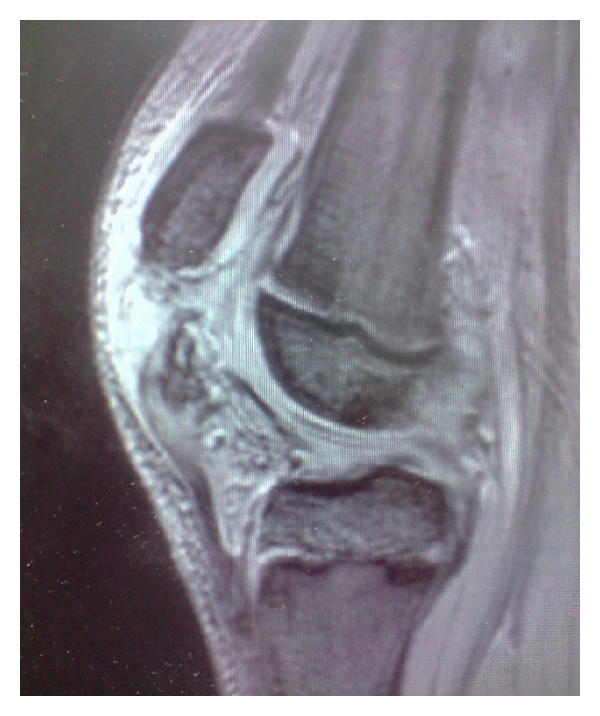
Sagittal T_2_ MRI of the right knee.
